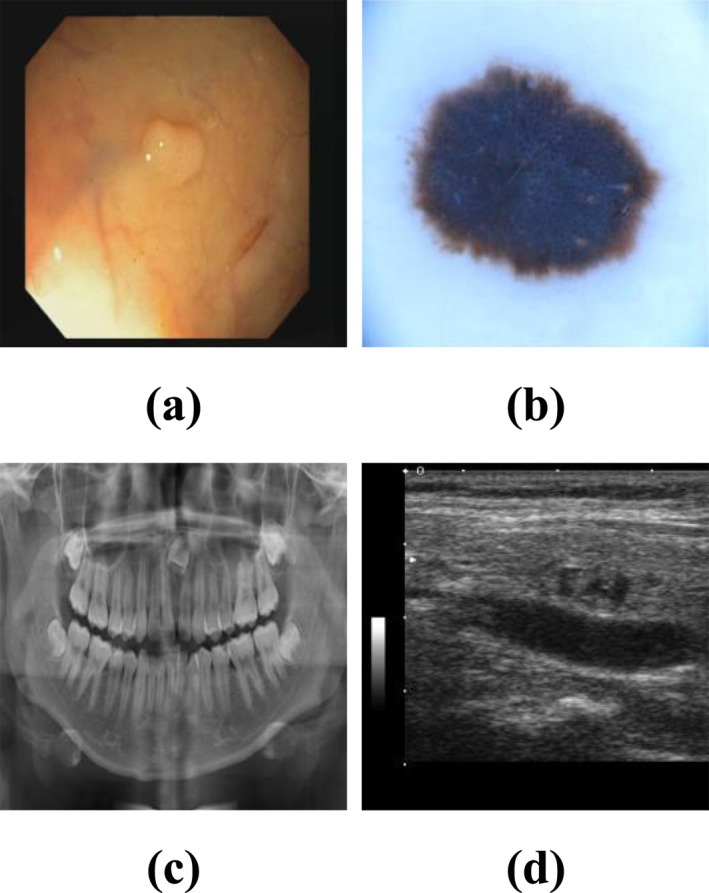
(a) A colonoscopy polyp image from the CVC‐ClinicDB dataset; (b) A dermoscopy lesion image from the ISIC 2017 dataset; (c) A dental x‐ray image from the MICCAI Tooth dataset; (d) A thyroid nodule ultrasound image from the DDTI dataset.
